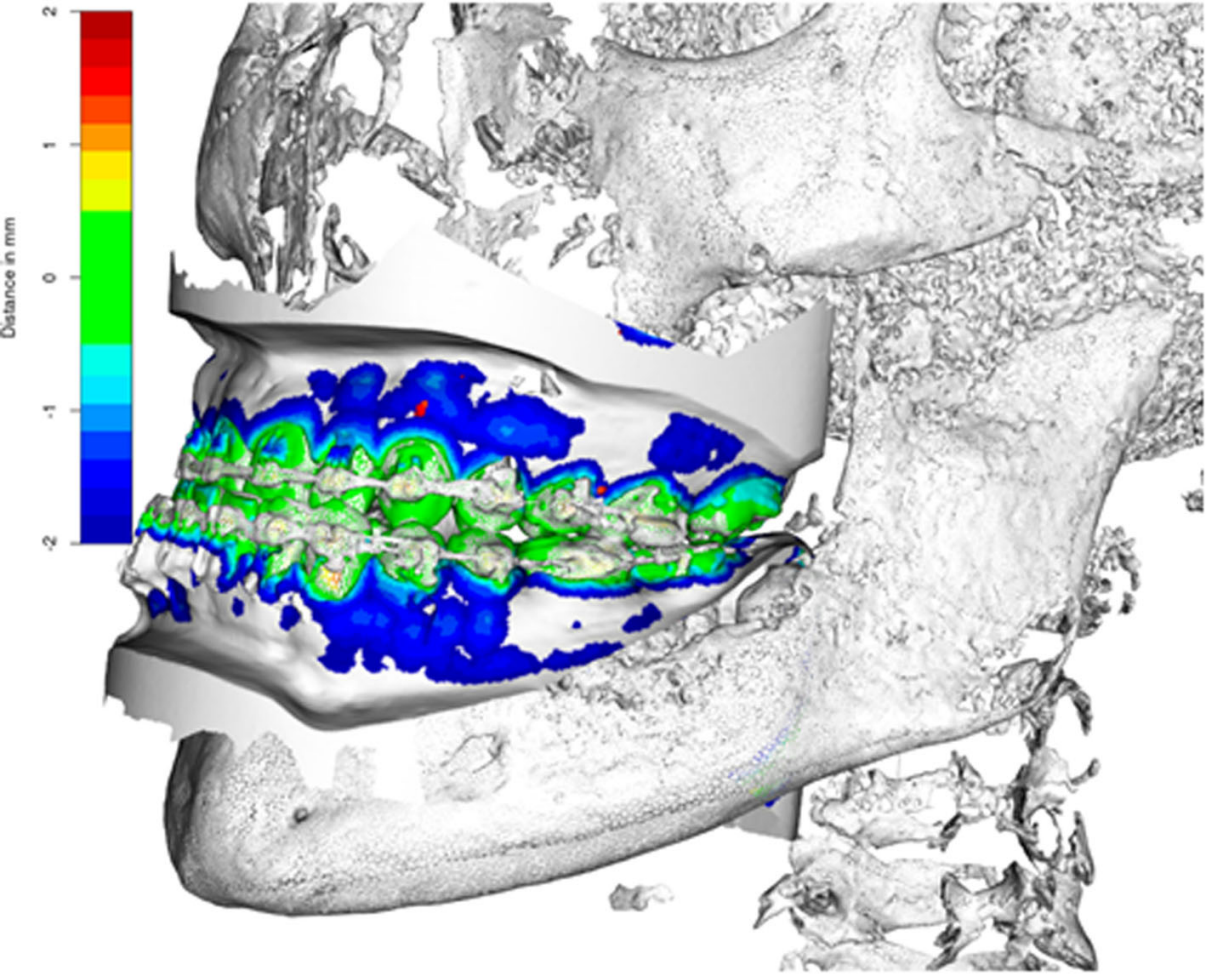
Superimposition of plaster cast and segmented CBCT scan. The deviation of the teeth surfaces after registration was throughout below 0.05 mm
